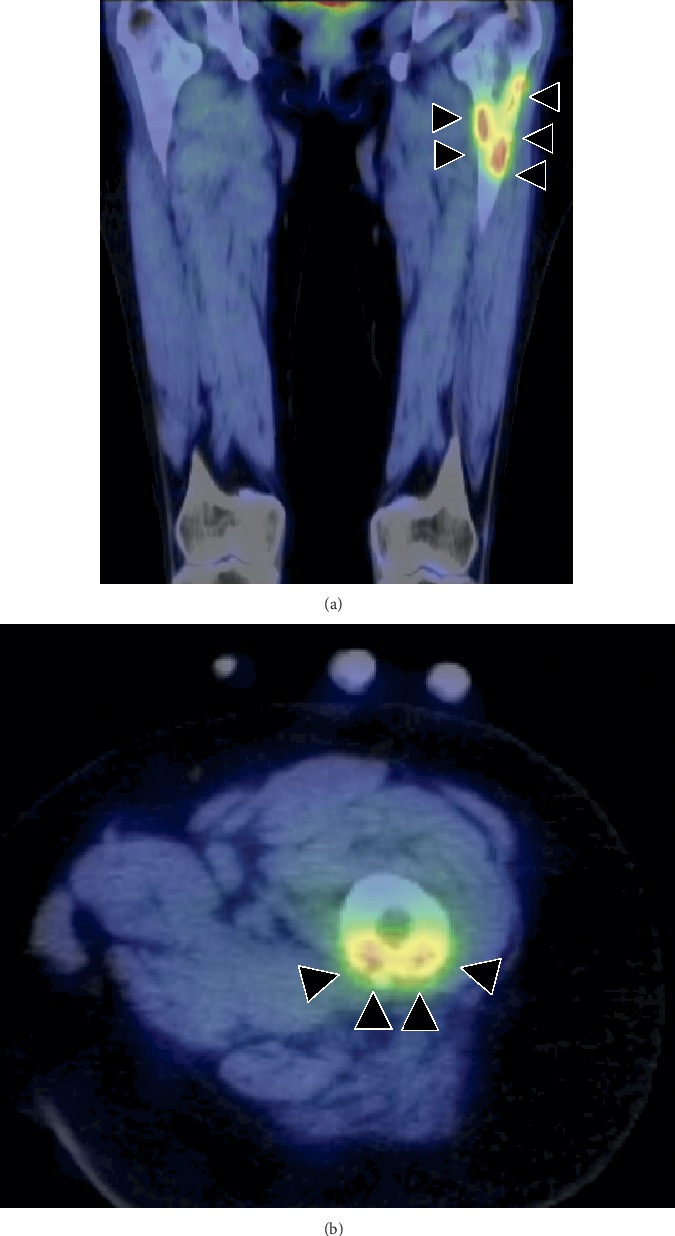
Whole-body PET/CT showing 18-FDG accumulations in the left femur only, suggesting a primary malignant bone tumor or chronic bacterial osteomyelitis (arrowhead). (a) Coronal view and (b) axial view.
